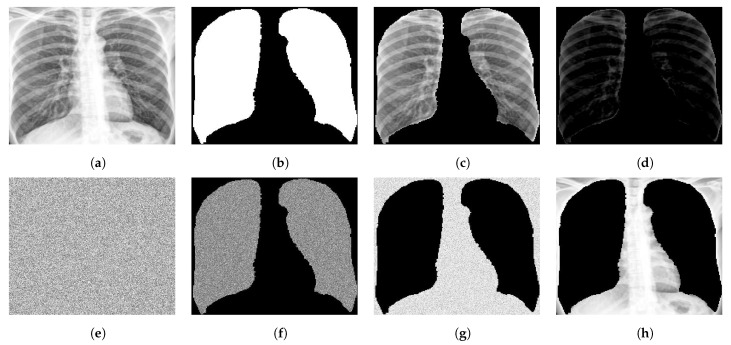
Different ways in which a chest X-ray image is modified to limit the information the classifier relies on. Top (left to right): (a) Cropped X-ray image, (b) lung mask, (c) segmented lung, (d) segmented lung with mean of background subtracted. Bottom (left to right): (e) Entire image randomized, (f) lung pixels randomized with non-lung area set to 0, (g) non-lung pixels randomized with lung pixels set to 0, and (h) lung pixels set to 0.
